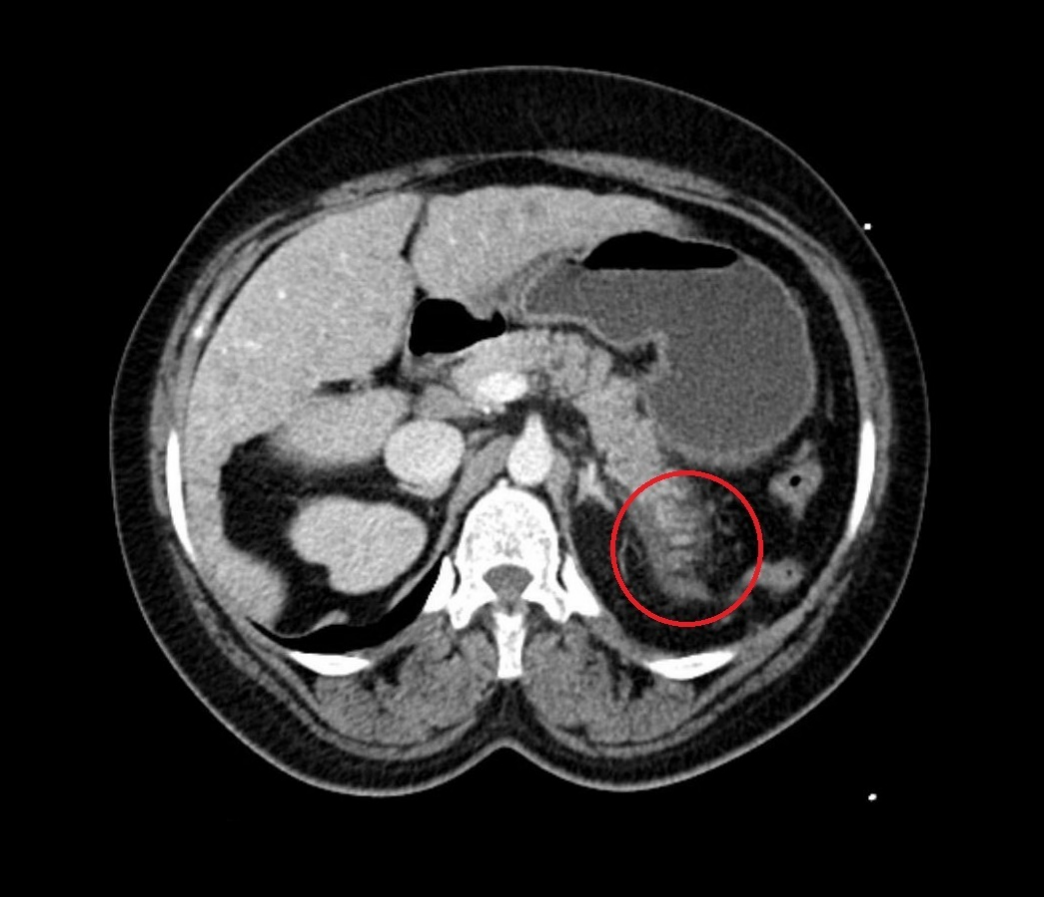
Computed tomography (CT) of abdomen showing fat stranding surrounding the pancreatic tail (red circle) consistent with acute pancreatitis.
